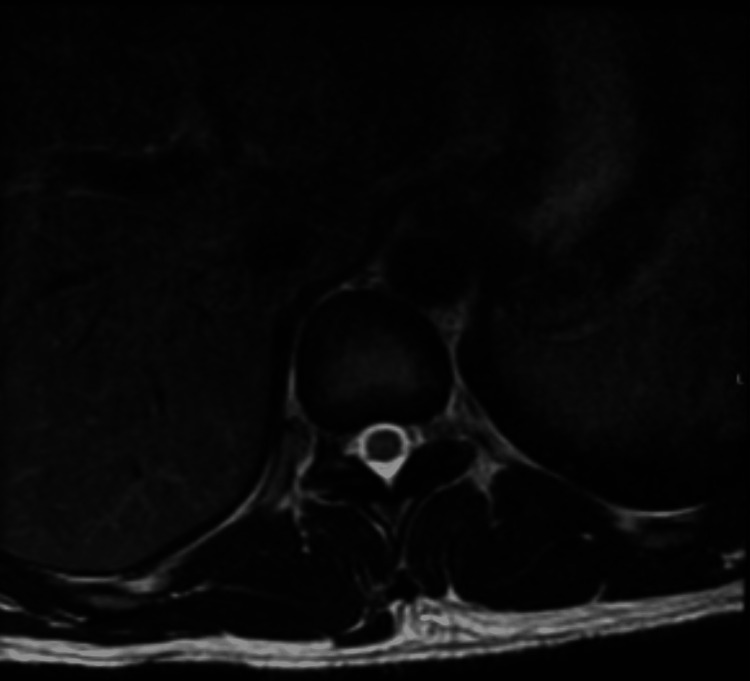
Thoracic spine MRI with contrast enhancement at the level of the conus medullaris on hospital day one showing unremarkable MRI.
